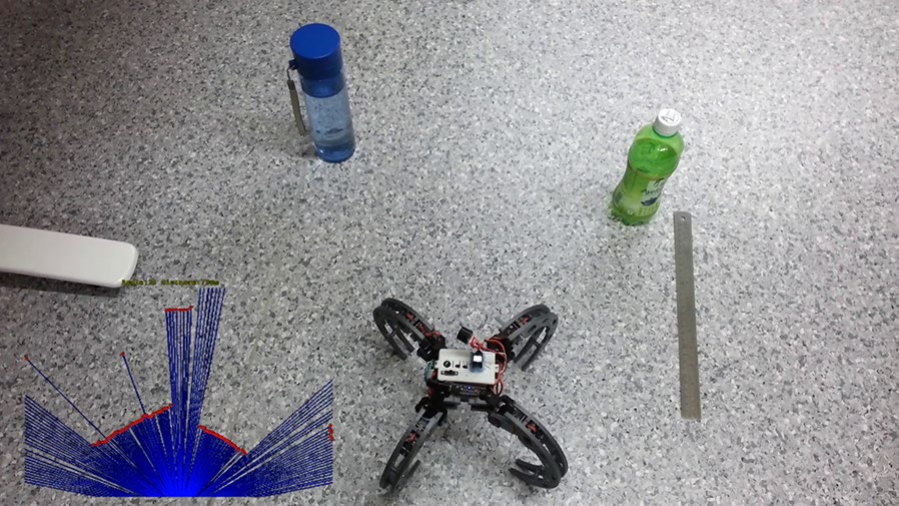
Sample ultrasonic scan. Sample scan with ultrasonic sensor
